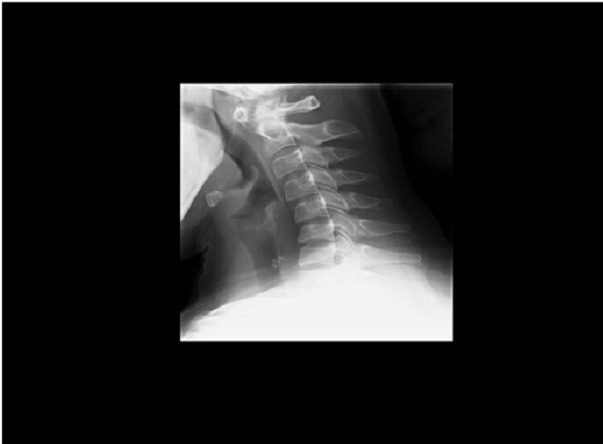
A lateral view of cervical spine radiograph showed an atloïdo-axoidien diastasis of 04 mm
